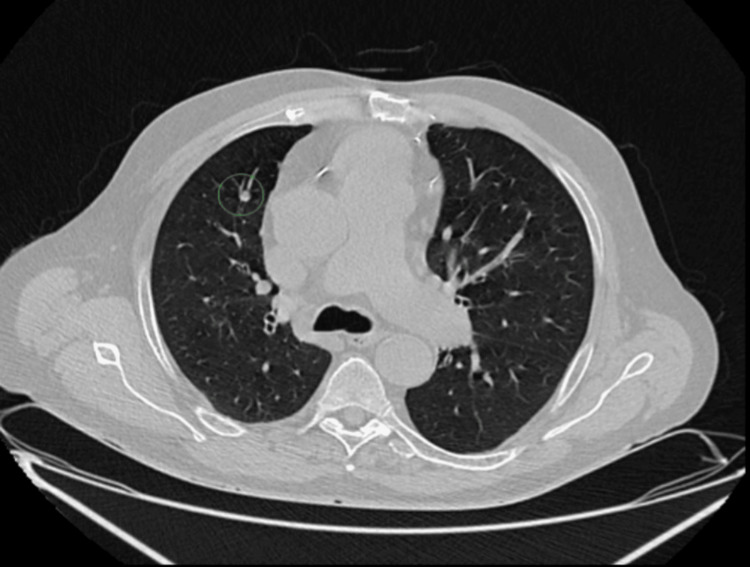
Axial chest CT scan without contrast shows solid nodule with regular and well-defined edges in the upper lobe of the right lung (indicated by green circle). Compared with previous CT scans dated September and January 2022, evidencing stability of the findings of previous results
